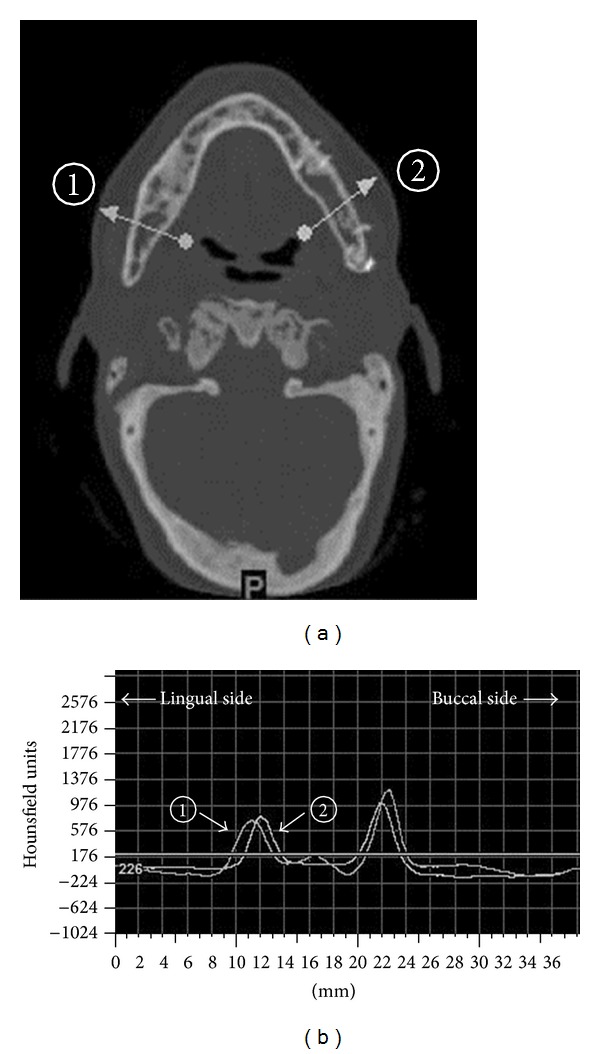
Bone density measurement using CT images and values [163]: (a) the regenerated bone shows no resorption 8 years postoperatively. The bone density was measured in 2 areas (one healthy bone area (1) and one regenerated bone area (2)) (arrows), and (b) the bone density. The peak of the cortical bone density was remarkably not different for the regenerative bone and the healthy bone.
